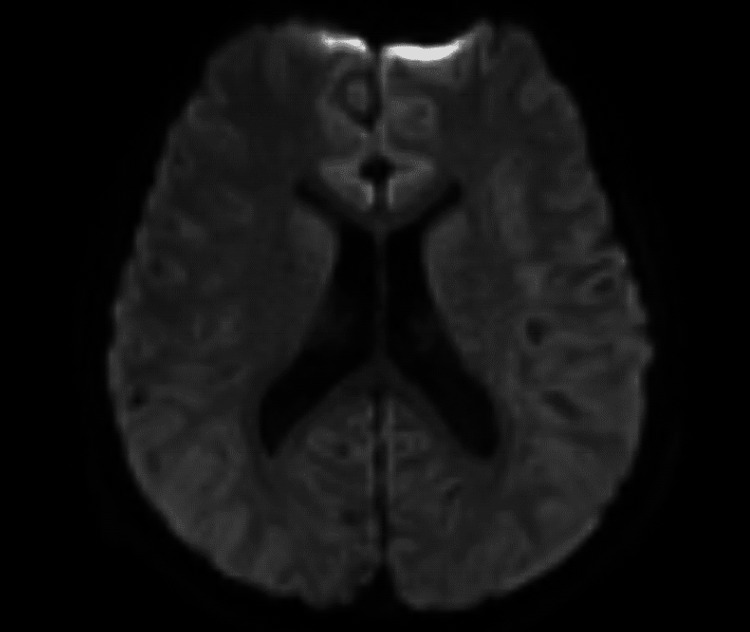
Brain MRI (axial diffusion-weighted images). There were no abnormal lesions.
